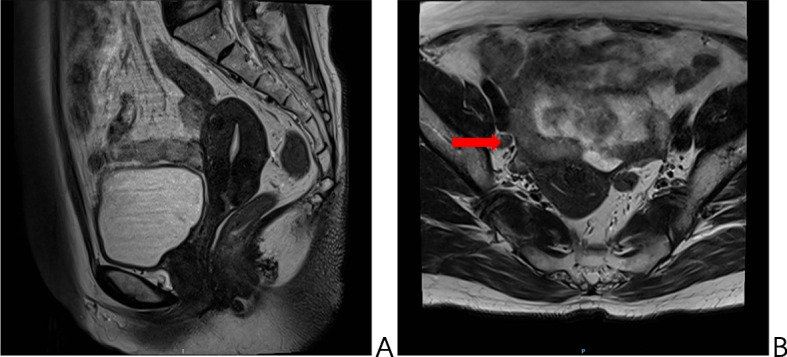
Post-radiotherapy (pre-surgery) MRI: A localised lesion significantly reduced. B enlarged lymph nodes on the right side (shown by arrows), insignificantly changed from before
